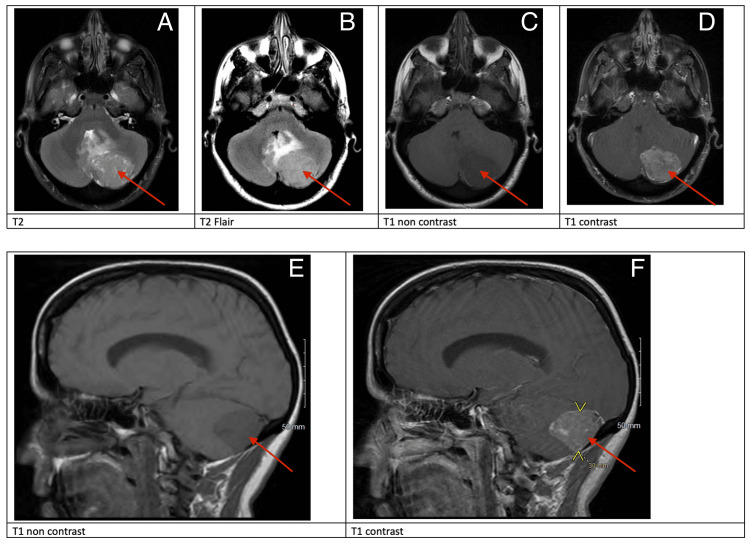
Preoperative MRI Red arrows: preoperative MRI demonstrating heterogeneous enhancement (C, D, E, F) and T2 (A) and T2 FLAIR (B) hyperintensity FLAIR: fluid-attenuated inversion recovery
